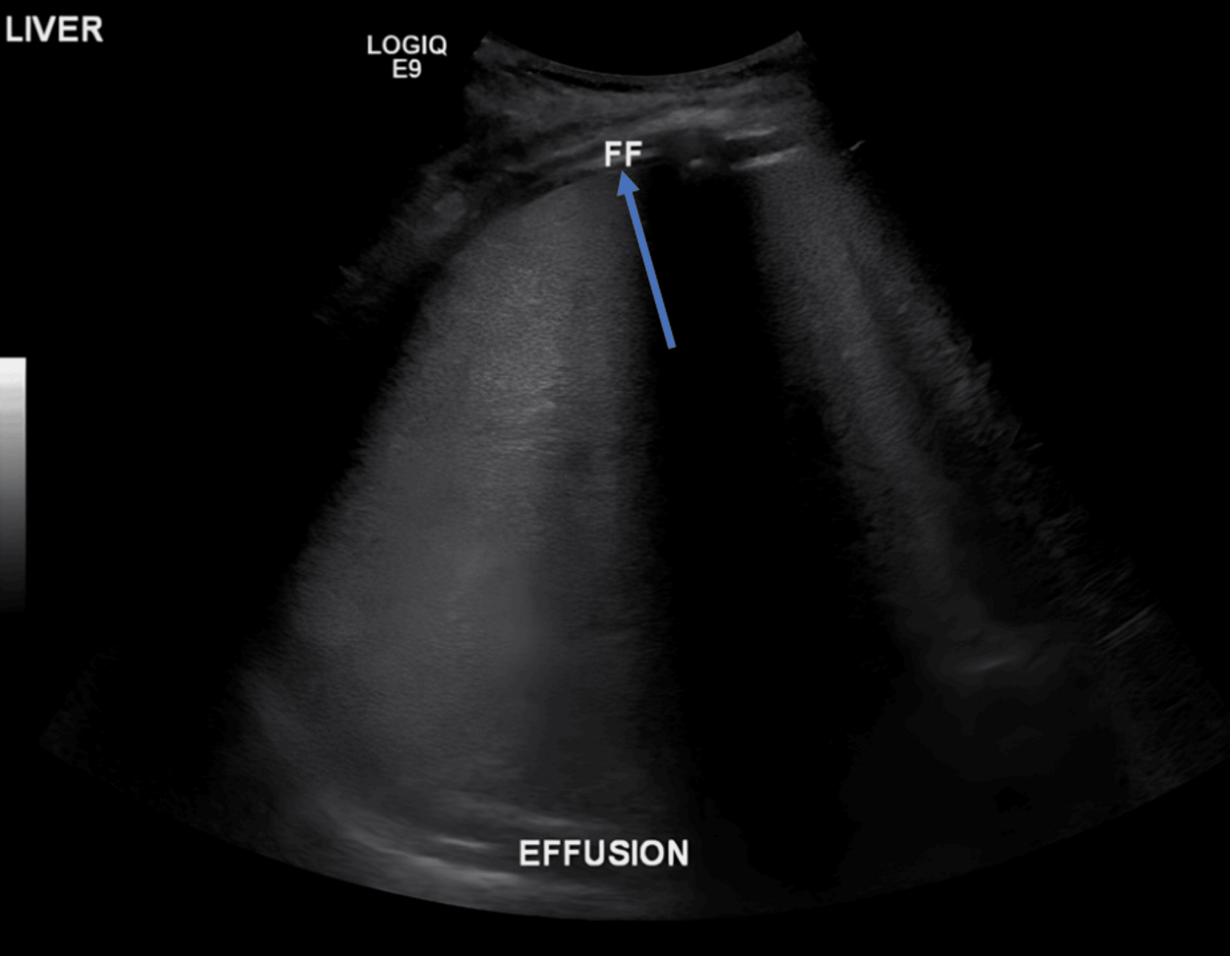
Chest ultrasound showing bilateral pleural effusion (blue arrow)
